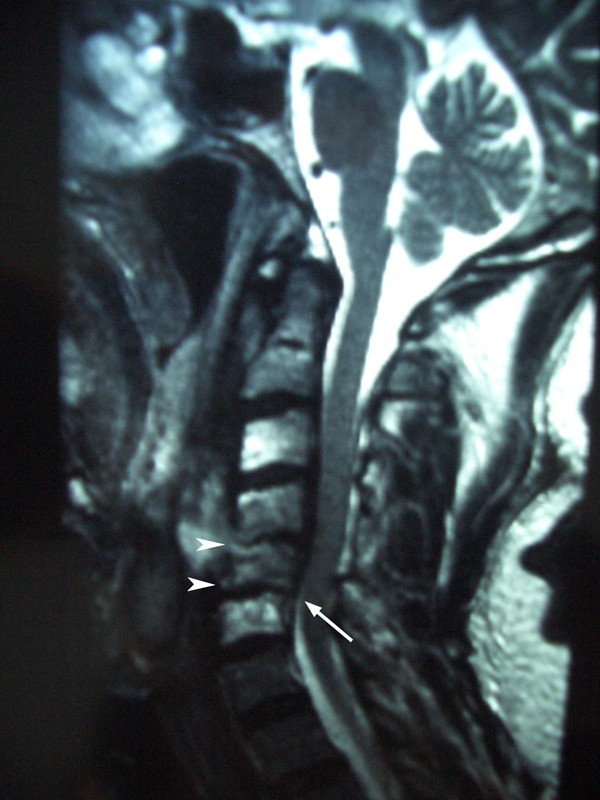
June 2000: MRI scan revealed a reduction of the disc height at C4-5 and C5-6 (arrow heads), a loss of height of the C5 vertebral body and retrolisthesis of C5 on C6 (arrow).
